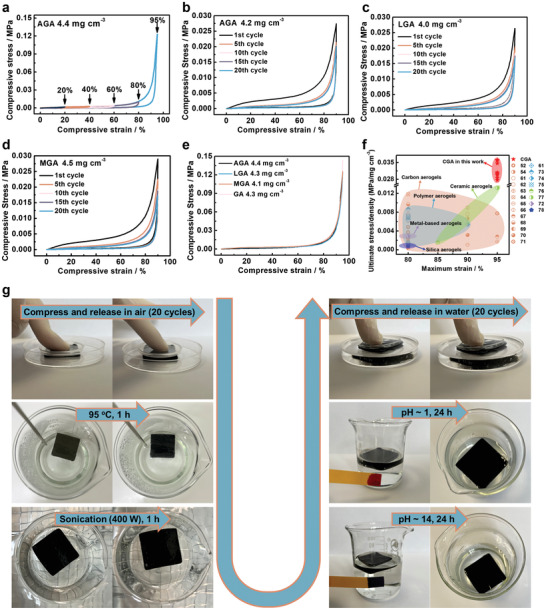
a) The stress‐strain curves of AGA at different compressive strains. The compressive stress‐strain curves of b) AGA, c) LGA, and d) MGA for 20 cycles of loading and unloading. e) Stress–strain curves of CGAs and GA at strain of 95%. f) Comparison of the normalized ultimate stress (compressive stress divided by density) of CGAs with other elastic aerogels as a function of the maximum strain. The detailed data for all the referred samples are listed in Table S3 (Supporting Information). g) The durability and stability of an AGA under a series of continuous harsh tests: 20 cycles of compression test in air, high temperature (95 °C, 1 h), ultrasonic agitation (400 W, 1 h), alkaline (pH ≈ 14, 24 h) environment, acidic (pH ≈ 1, 24 h) environment, and 20 cycles of compression test in water.
